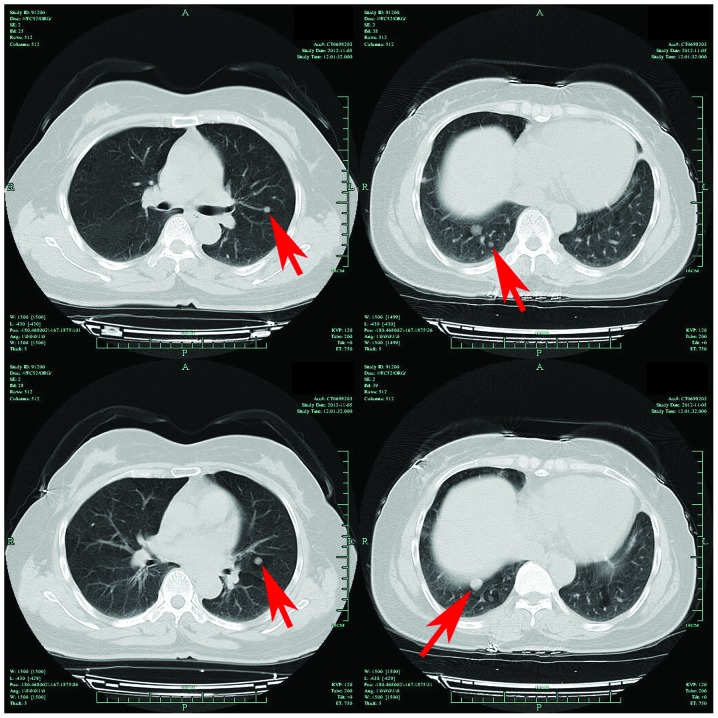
Contrast-enhanced computed tomography of the chest revealed multiple round pulmonary nodules, measuring ~10×12 mm with clear boundaries, in the lungs (indicated by the arrows).
